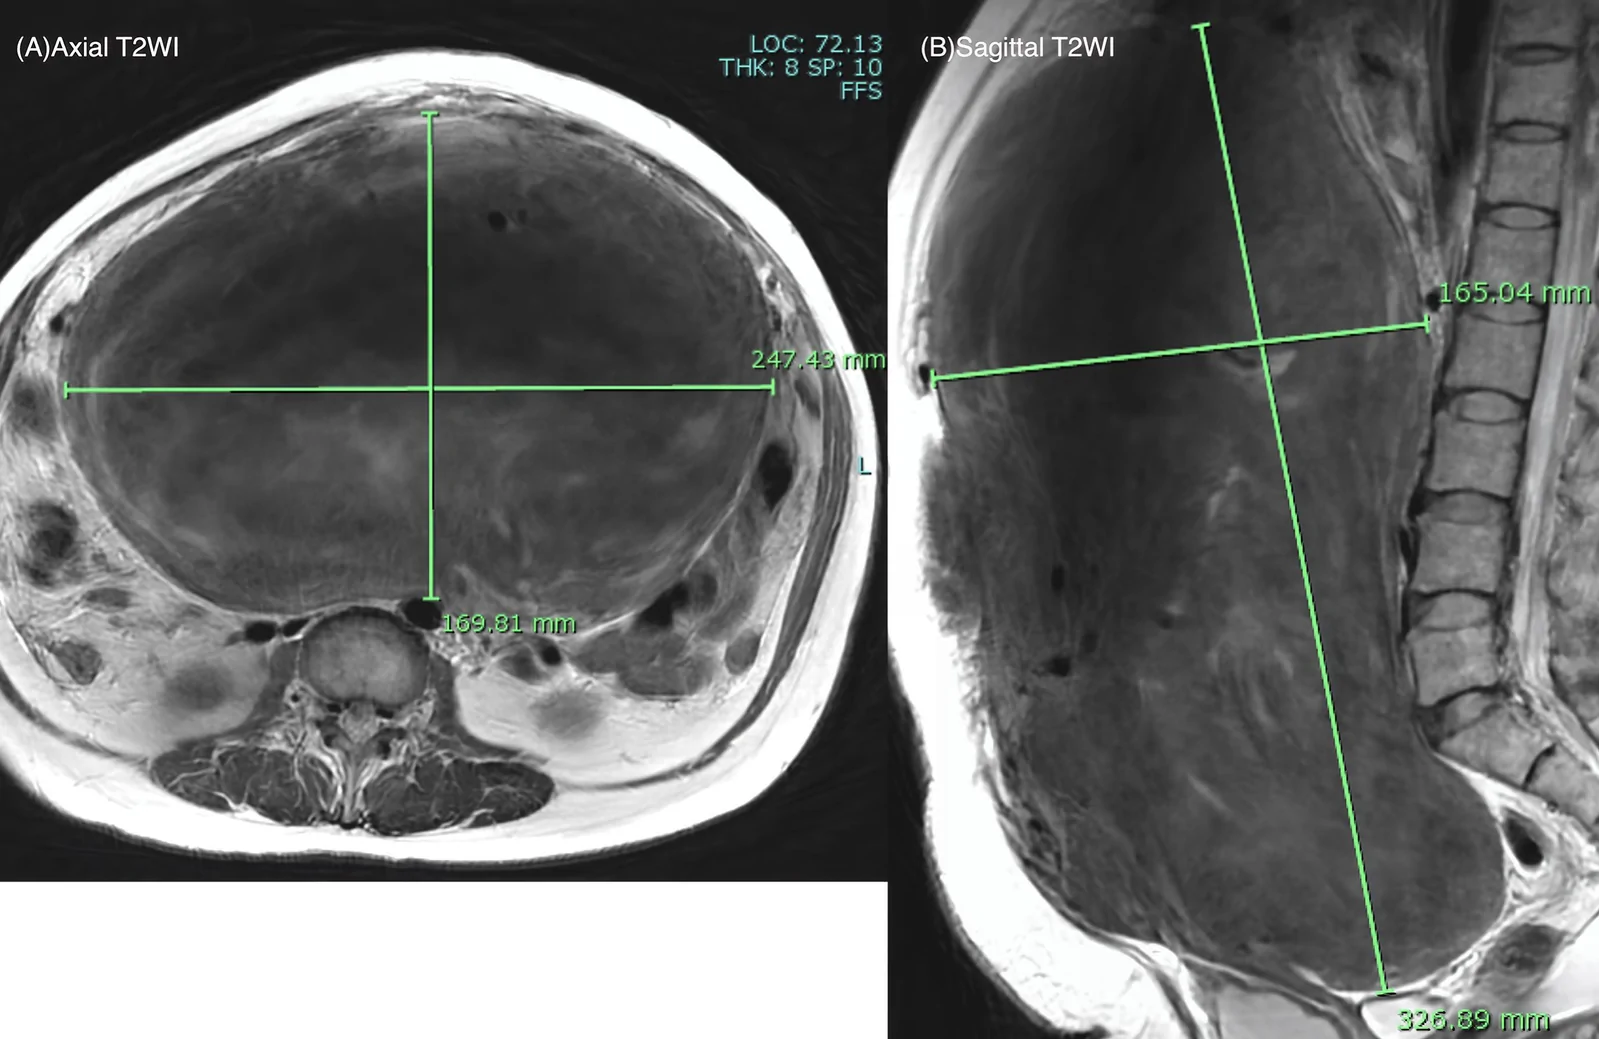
Preoperative magnetic resonance imaging findings. T2-weighted images showing a giant uterine mass with heterogeneous signal intensity, measuring approximately 32.7 × 24.7 × 17.0 cm. (A) Axial view. (B) Sagittal view.
